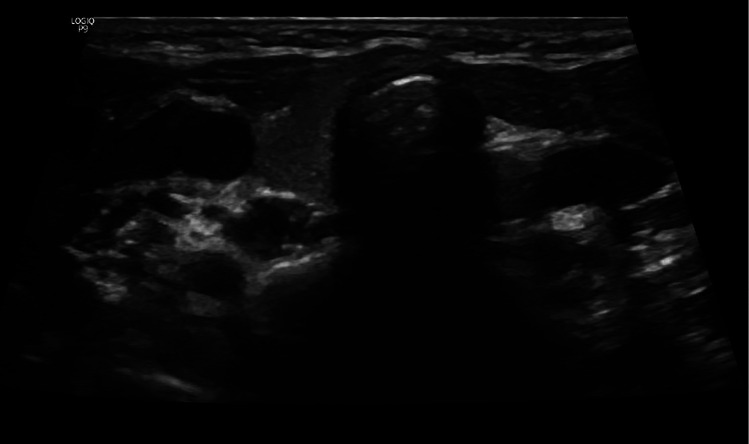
Neck ultrasound showing a non-visualized left thyroid lobe.
